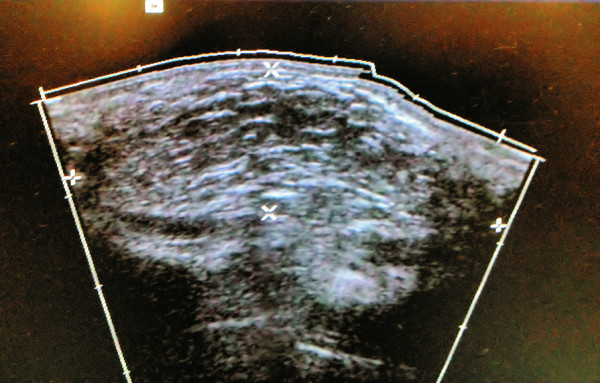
Preoperative ultrasound scan of the tumor showed a well circumscribed isoechoic well defined mass.
